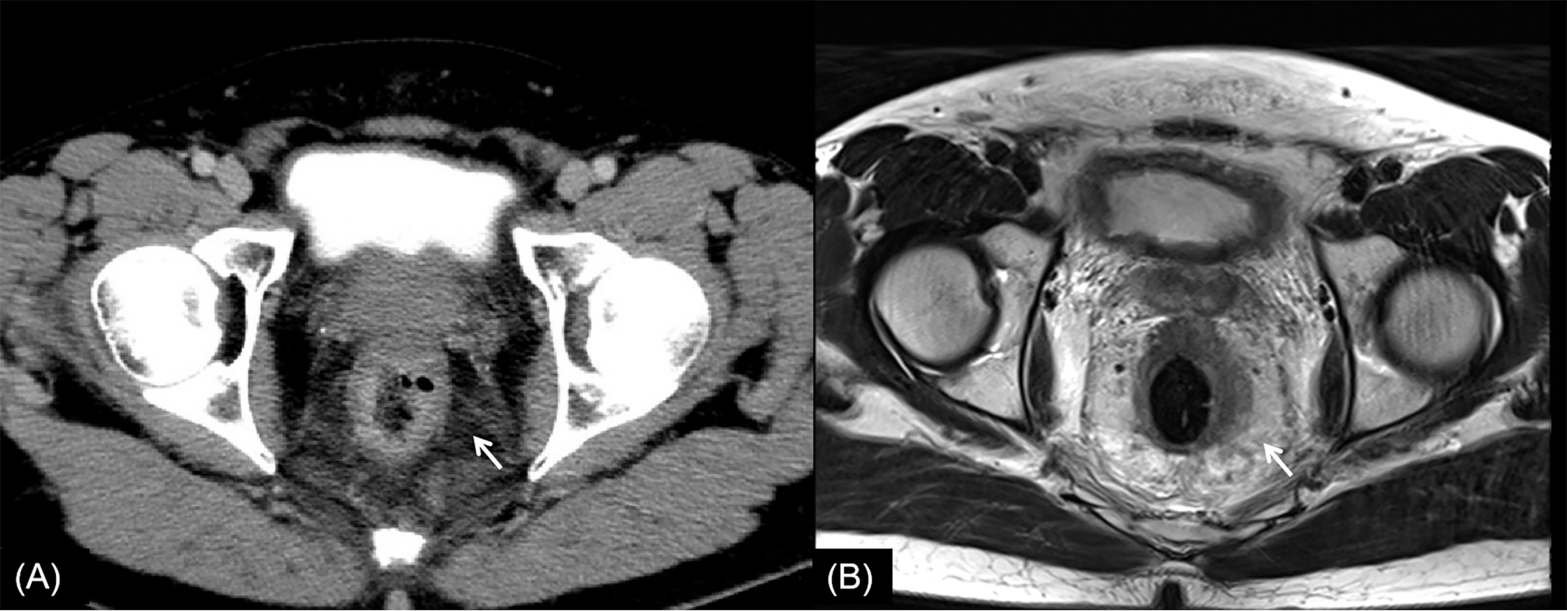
Rectal cancer with ypT3N2. (A) Axial MSCT depicts left rectal wall thickening and mesorectal edema (arrow). Accurately restaged as T3. (B) MRI transverse high-resolution T2WI shows thickened left rectal wall involving mesorectal fascia (arrow). Accurately restaged as T3. No enlarged lymph nodes were found in MSCT images. Low restaged as N0 with MSCT and MRI. yp, pathological staging after neoadjuvant chemoradiotherapy; T, tumor; N, node; MSCT, multi-slice computed tomography; MRI, magnetic resonance imaging; T2WI, T2-weighted imaging.
